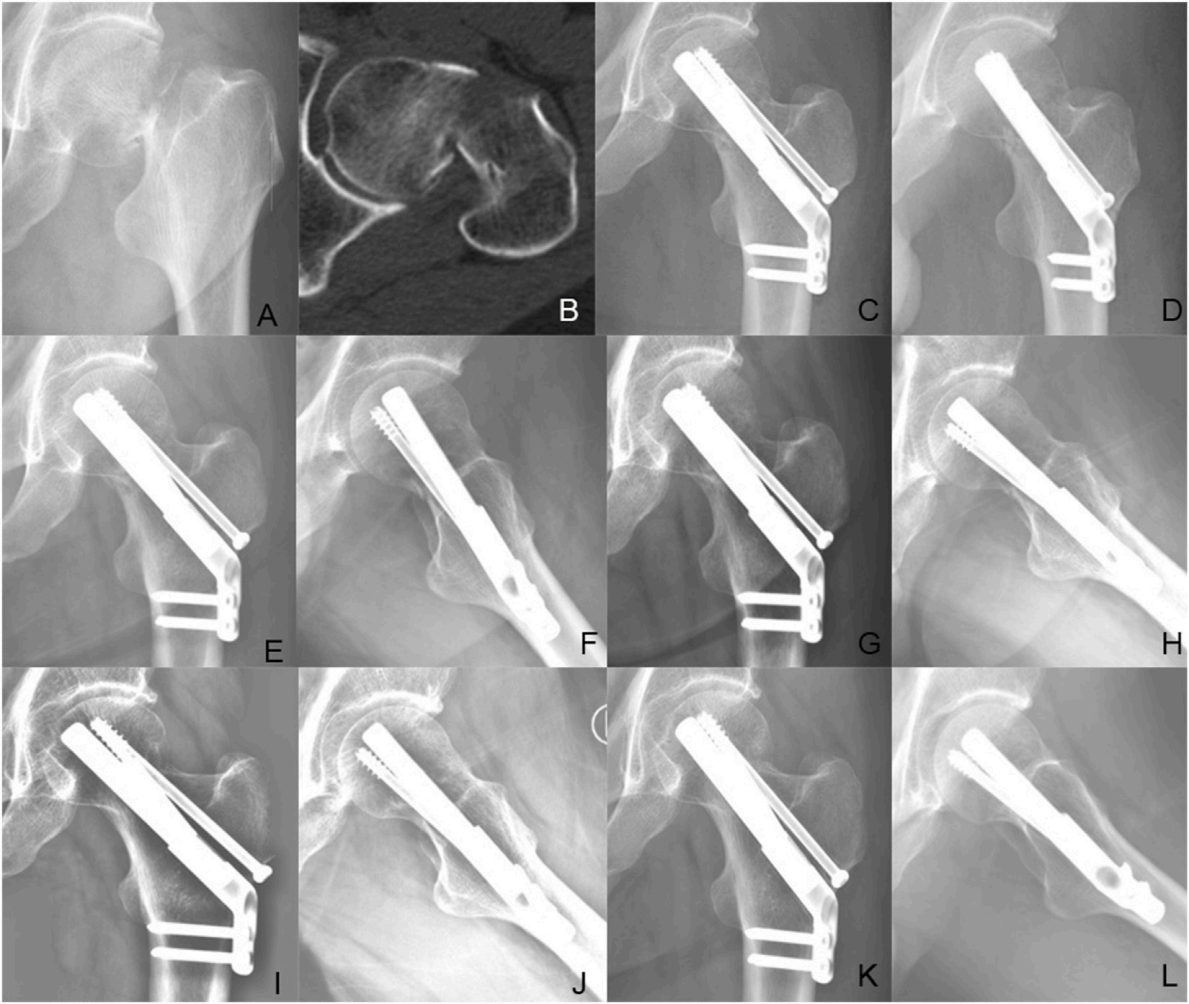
Case presentation: a 56 years old man with left femoral neck fracture fixed with FNS + CS. (A,B) Preoperative anteroposterior radiograph and CT scan showed the right FNF with posteromedial cortex fracture. (C,D) The anteroposterior and lateral X-ray with FNS 1 day after surgery; (E,F) The anteroposterior and lateral X-rays 1 month after surgery; (G,H) The anteroposterior and lateral X-rays 3 months after surgery; (I,J) The anteroposterior and lateral X-rays 6 months after surgery; (K,L) The anteroposterior and lateral X-rays 12 months after surgery.
